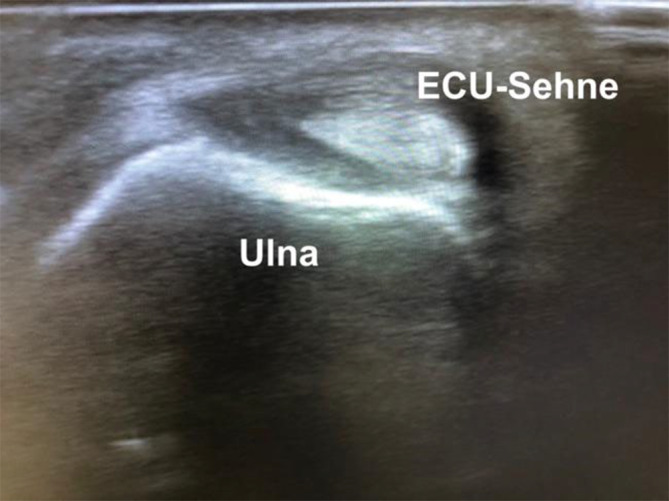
Bei einer Instabilität im DRUG ist eine CT(Computertomographie)-Untersuchung in Pro- und Supination empfohlen, wobei diese bei der fortgeschrittenen Arthrose vermutlich entbehrlich ist [12, 14].
